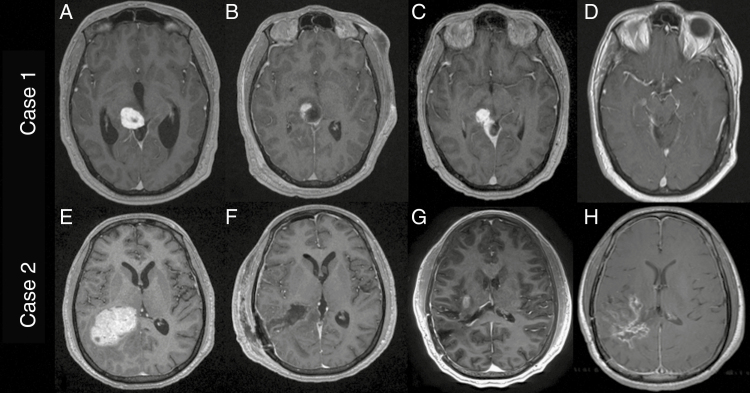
Case 1: T1 post-contrast MRI images of case 1 demonstrating initial tumor (a), immediate post op (b), recurrence at 3 months (c), and 52 months after SRS showing no progression (d). Case 2: T1 post-contrast MRI images of case 2 demonstrating pre-op (a), post-op (b), recurrence at 11 months (c), and stable disease burden on crizotinib monotherapy at 23 (d)
